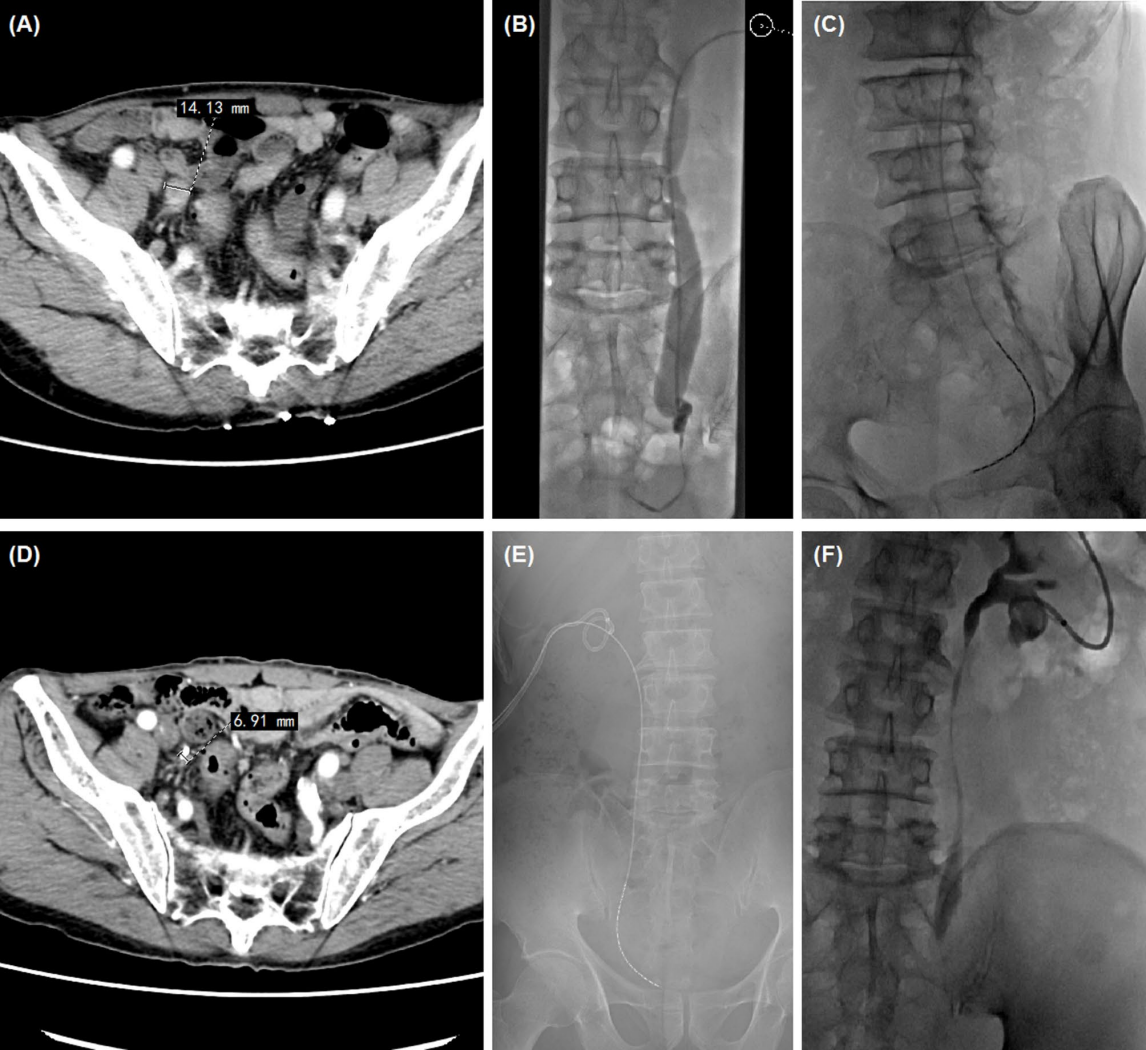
A 67-year male treated by iodine-125 seed strand for ureteral carcinoma. (A) CT revealed ureteral carcinoma in the right ureter, with a diameter of 14.13 mm. (B) Urothrography revealed a segmental occlusion in the lower segment of right ureter. (C) A Iodine-125 seed strand with 20 seeds was inserted. (D) CT revealed a significant shrunk tumor (6.91 mm in diameter) after 2.2 months’ follow-up. (E-F) The iodine-125 seed strand was removed after 6.8 months, and normal urothrography was shown
